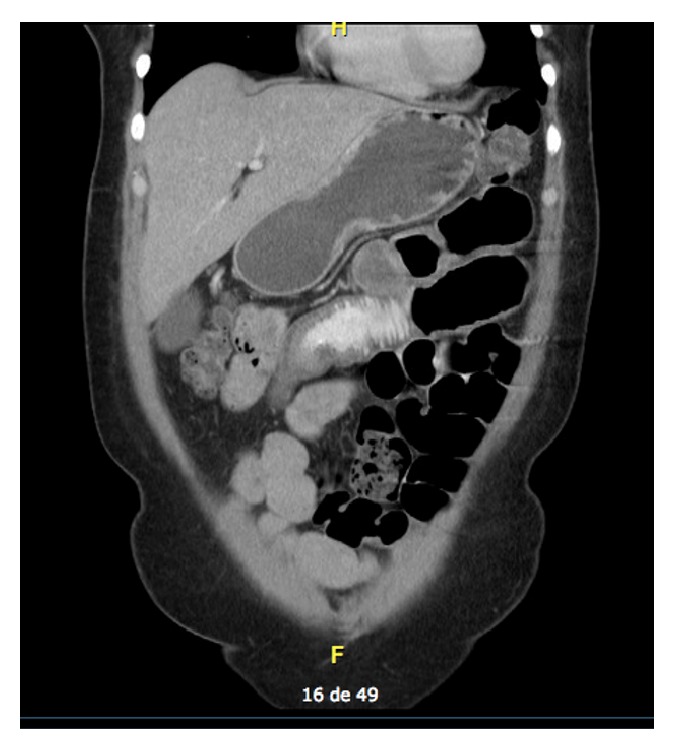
CT imaging presenting adynamic ileus with jejunal loop thickening.
